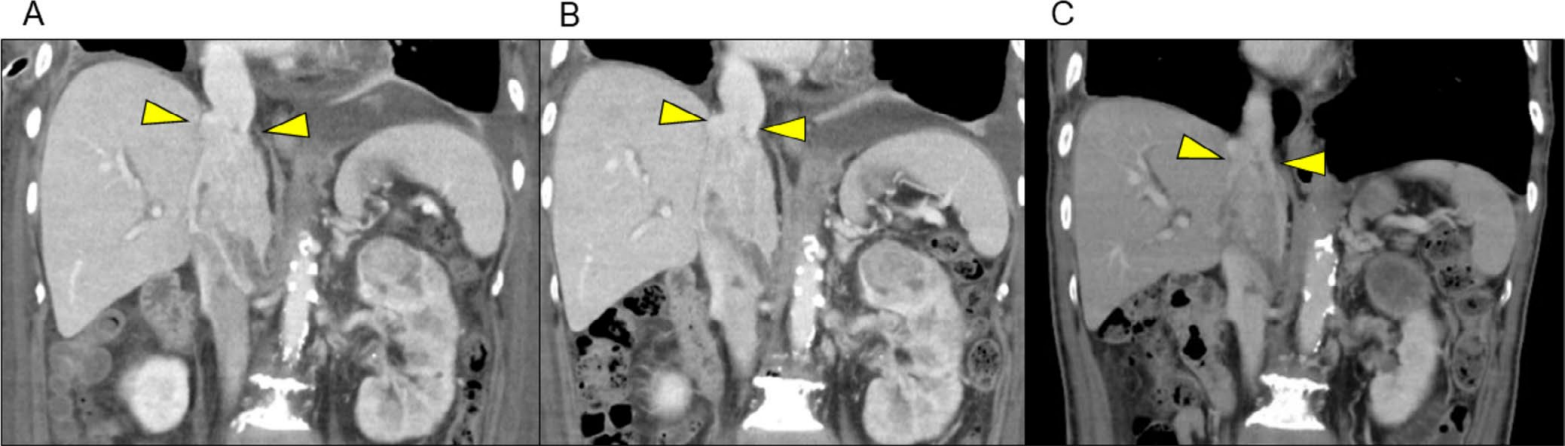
Sequential tumor evaluation following emergency surgery, as shown in coronal CT images. A, B, and C were taken 26, 41, and 67 days postoperatively, respectively. Axitinib was resumed at time (B). Slight reduction of the IVC thrombus and primary renal tumor was observed, with the cranial end of the IVC tumor near the hepatic vein inflow at the time of nephrectomy.
